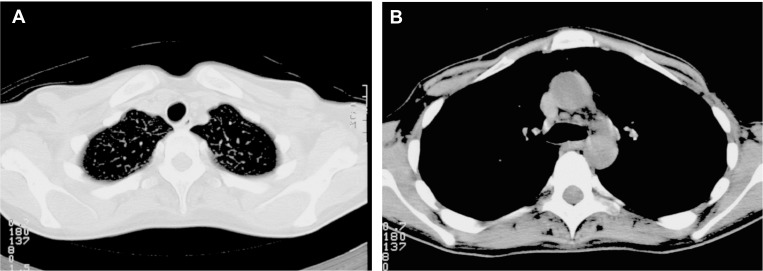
Axial computed tomography (CT) scan in June 1996, two months after high-dose chemotherapy and consolidation radiation A. The CT scan shows no detectable tumor in the lung. B. The CT scan shows no detectable mass in the aortic pulmonary window.
